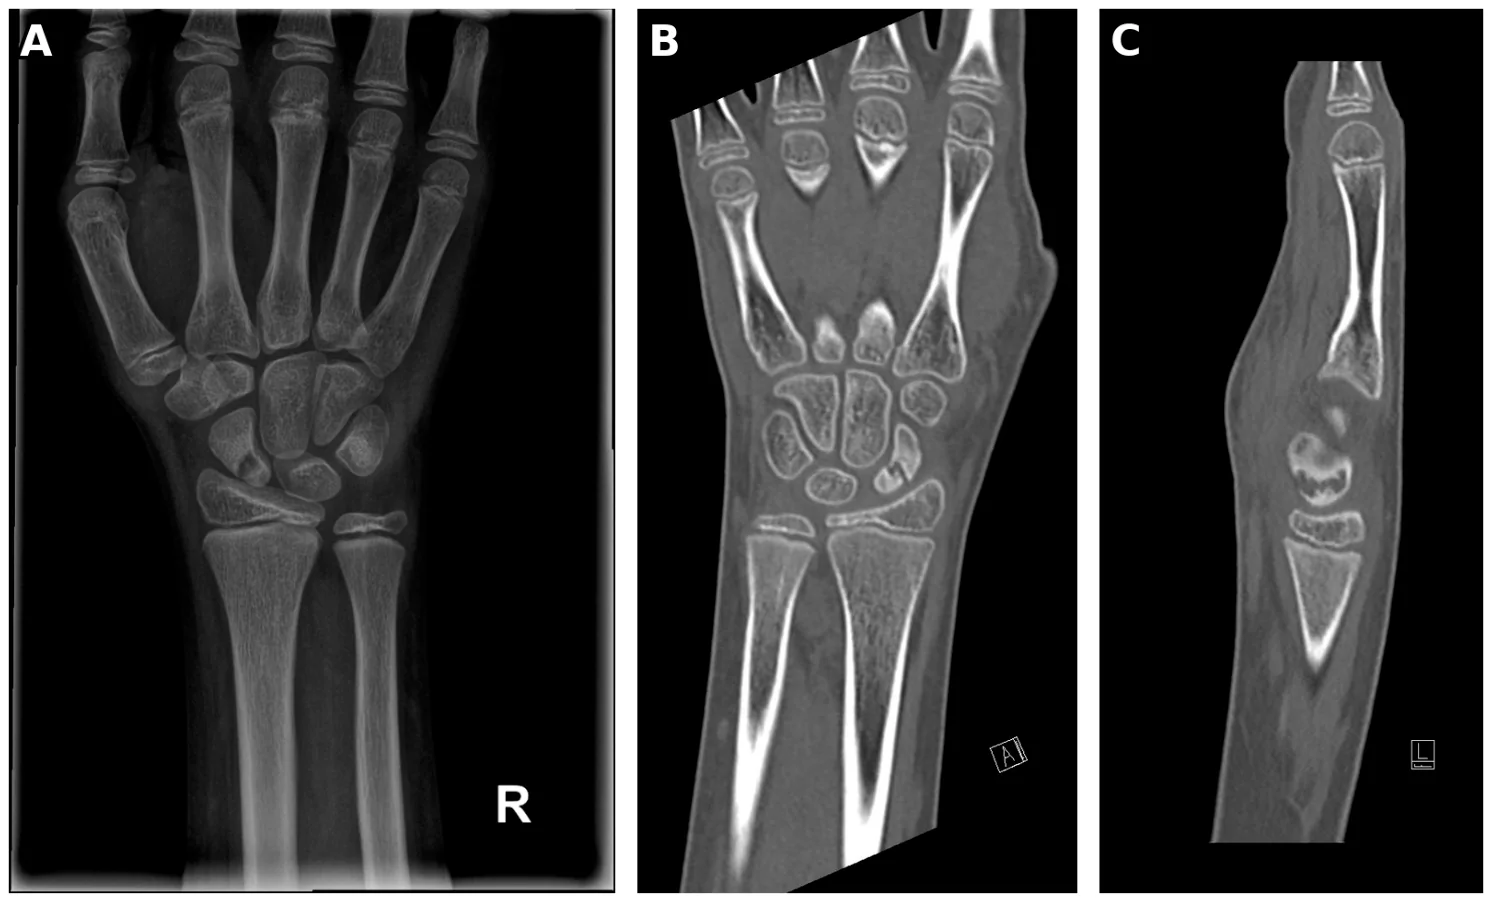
Diagnostic imaging of the inferior-pole scaphoid nonunion. (A) Initial posteroanterior radiograph retrospectively showing a nondisplaced or minimally displaced inferior-pole fracture that was missed at the initial emergency-department visit. (B) Coronal and (C) sagittal CT reconstructions obtained at referral, demonstrating a persistent fracture line, corticated margins, interfragmentary separation, and absence of bridging trabeculae.
